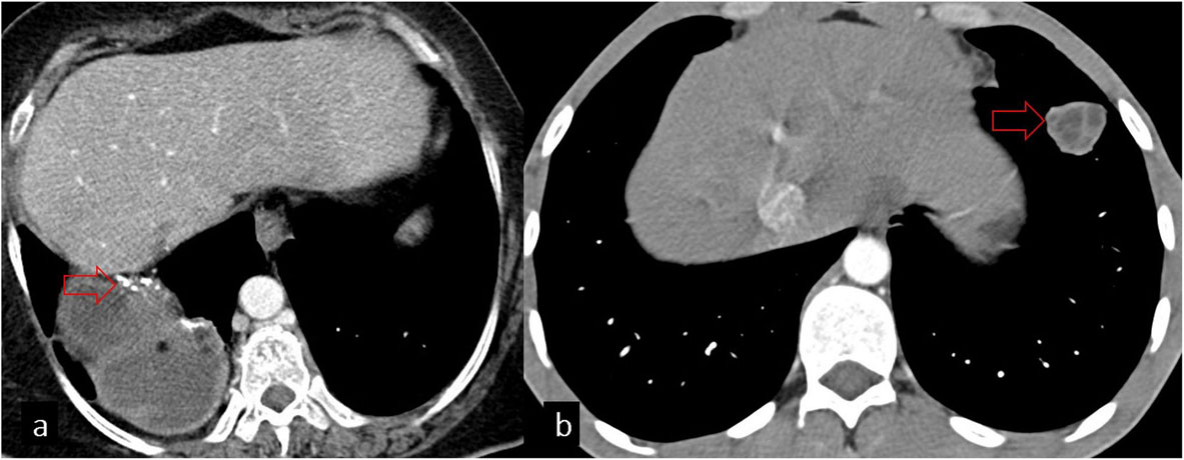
a Calcification in the wall of the hydatid cyst is seen on the axial CT image (red arrow). b Daughter cysts in the hydatid cyst (red arrow)
